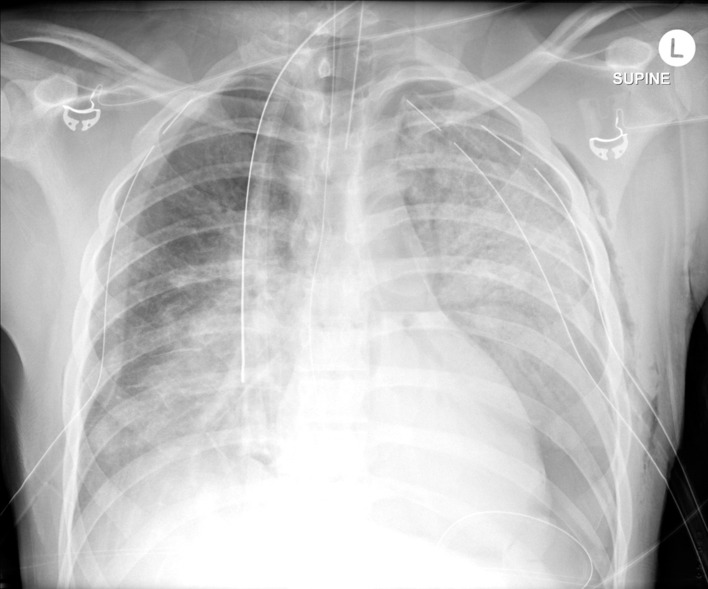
Chest x-ray showing bilateral pulmonary contusions and pneumothoraxes requiring chest tube placement.
